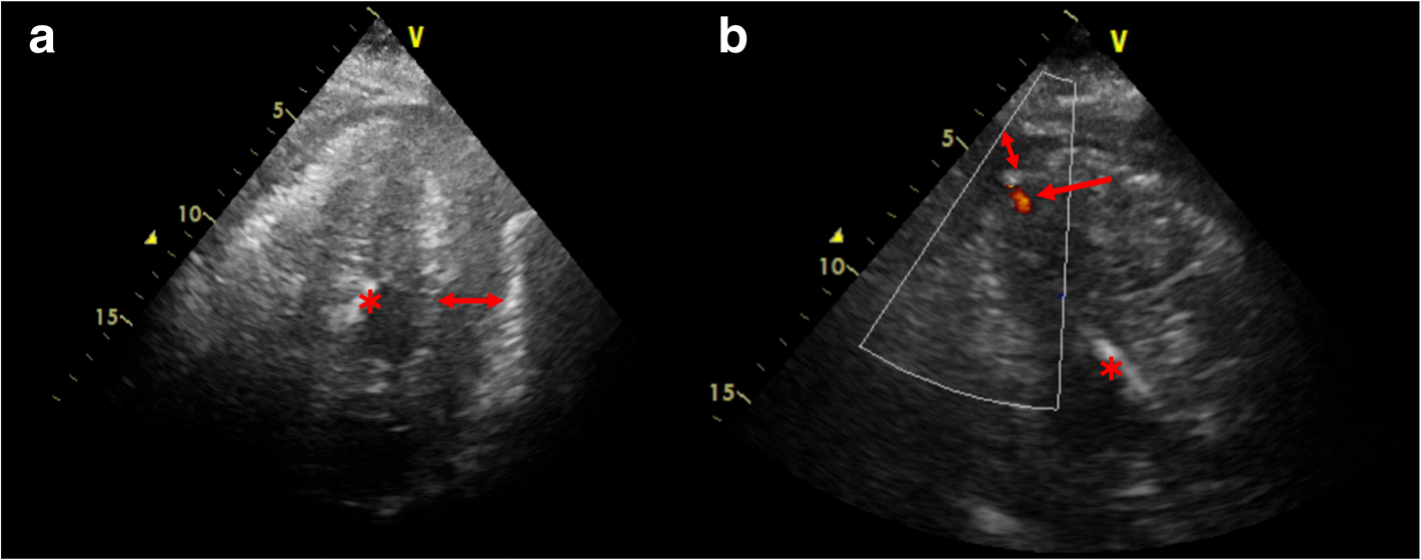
Apical four chamber view (a) and apical long-axis view (b) by transthoracic echocardiography. Note the pericardial effusion (double arrow) and the abnormal flow on apex of the left ventricle (small arrow), part of the IMPELLA® (asterisk). The tip of the IMPELLA® is not depicted
